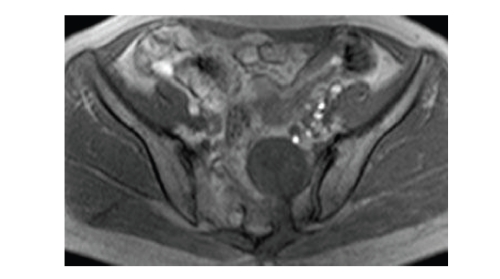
Axial T1-weighted MR image demonstrates dumbbell-shaped tumor
